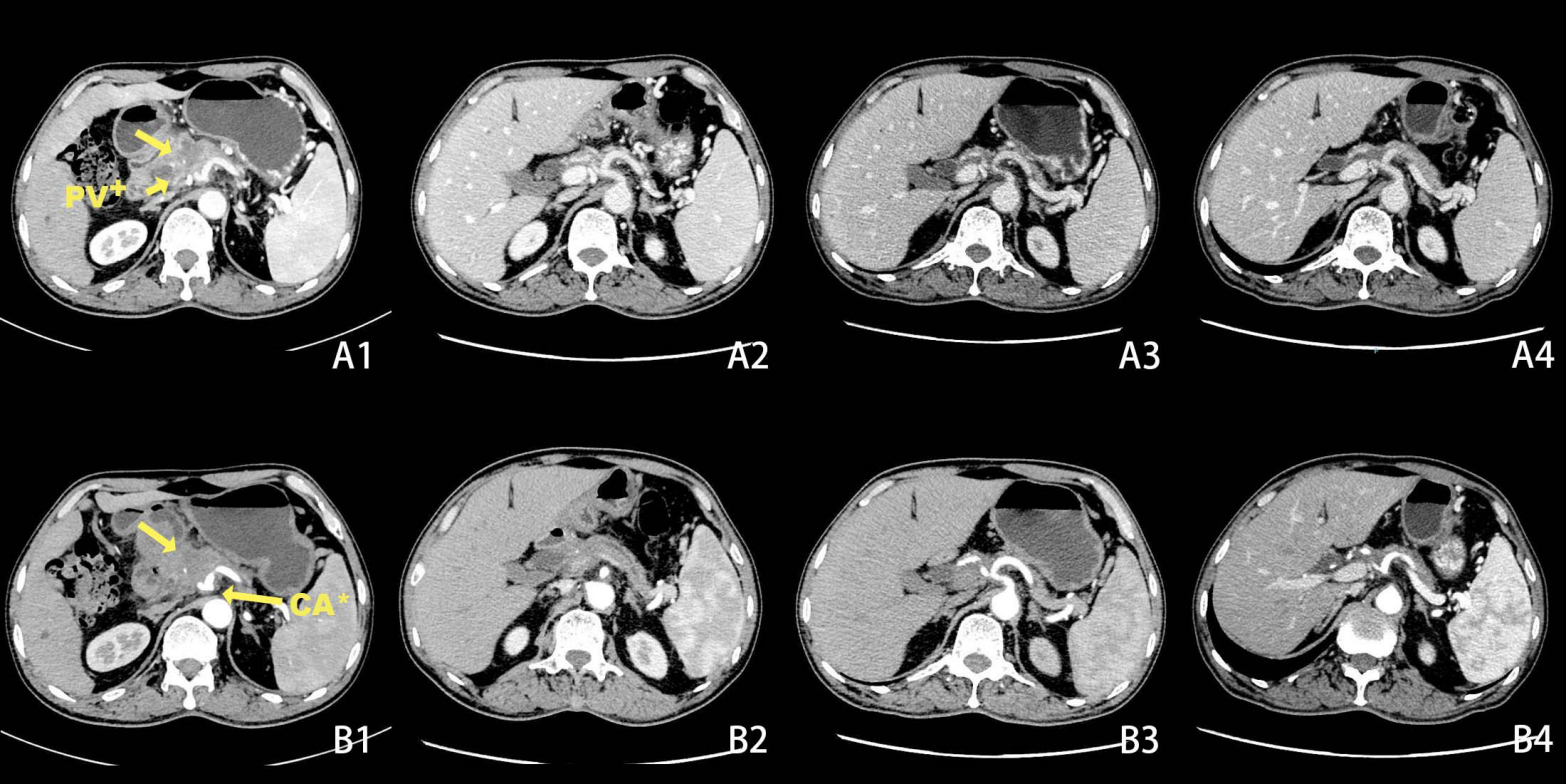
Changes in tumor size before and after chemotherapy on CT scans + Portal Vein, * Coeliac Axis. Before chemotherapy CT scan of the abdomen shows a lesion in the pancreatic neck with portal vein and coeliac axis invasion (A1, B1). After 2 cycles of chemotherapy, the tumor had significantly decreased in size (A2, B2). After 5 cycles of chemotherapy (A3, B3). After 6 cycles of chemotherapy(A4, B4).
